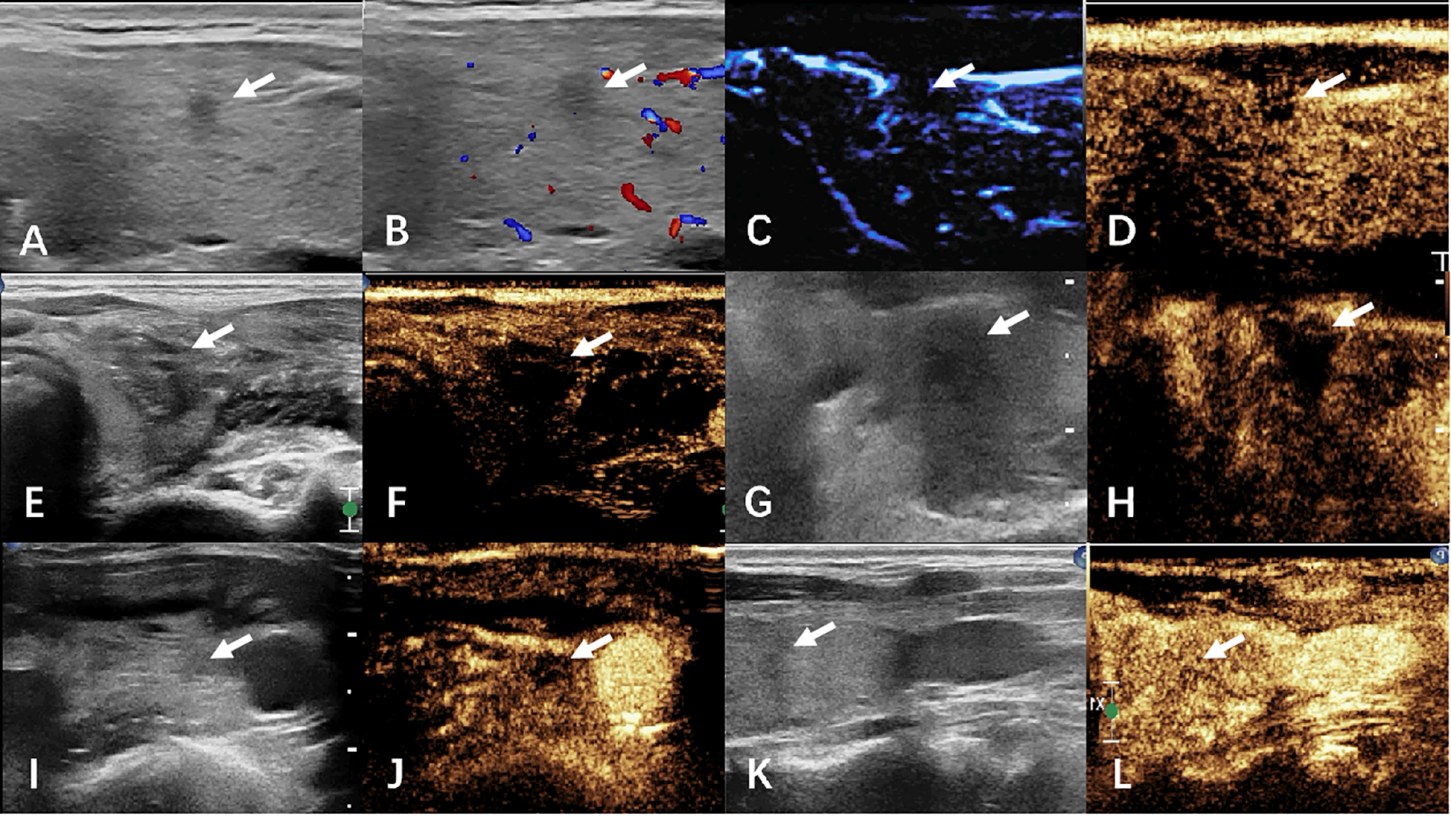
The images of CEUS group before and after ablation were compared. (A–D) preoperative ultrasound, CDFI, MFI, and CEUS. (E, F) Ultrasound and CEUS immediately after ablation. (G, H) ultrasound and CEUS at 1 month after surgery. (I, J) Ultrasound and CEUS at 6 months after surgery. (K, L) Ultrasound and CEUS at 12 months after surgery. White arrows indicate lesions.
